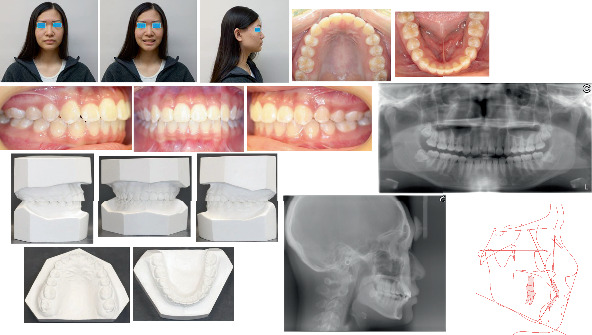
Posttreatment extra- and intraoral photographs, models, radiographs, and cephalometric tracing (red).
